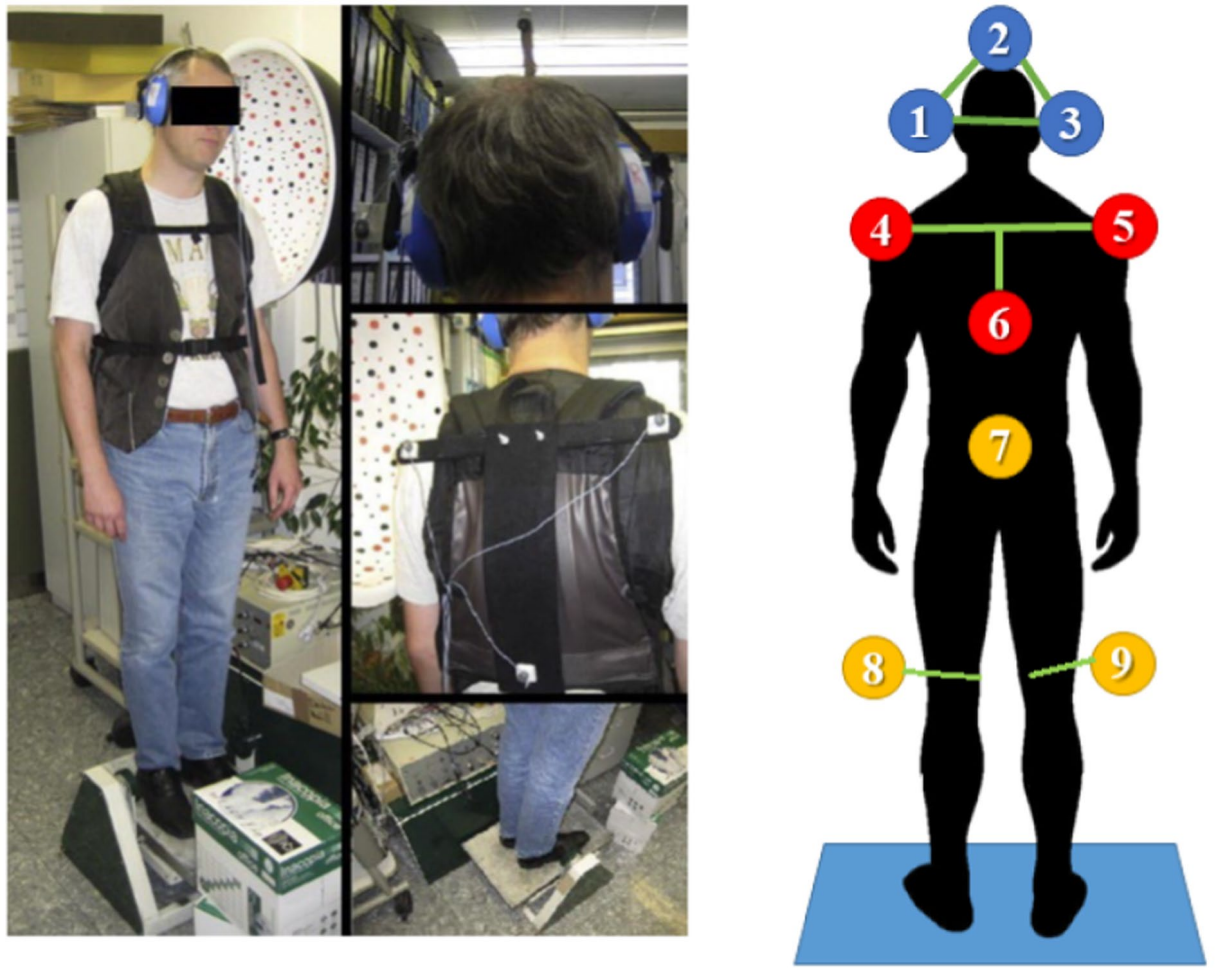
Left: The experimental setup. The test subject stands on a platform, tilting in the sagittal plane around the ankle. The subject’s feet were placed within marked positions, with the heels together and the tips spread 15° apart, while the arms hung loosely by the sides. The tilt axis of the platform was ensured to act through the anatomical upper ankle joint of the subject. Right: 3D ultrasound position markers (Zebris system) placement. Head (1, 2, 3), upper chest (4, 5, 6), hip (7), and lateral femoral epicondyles (8, 9). Pictures from (3). Patients were in their everyday clothing including shoes and on regular medication in dopaminergic “ON,” to reflect conditions in which most falls occur.
